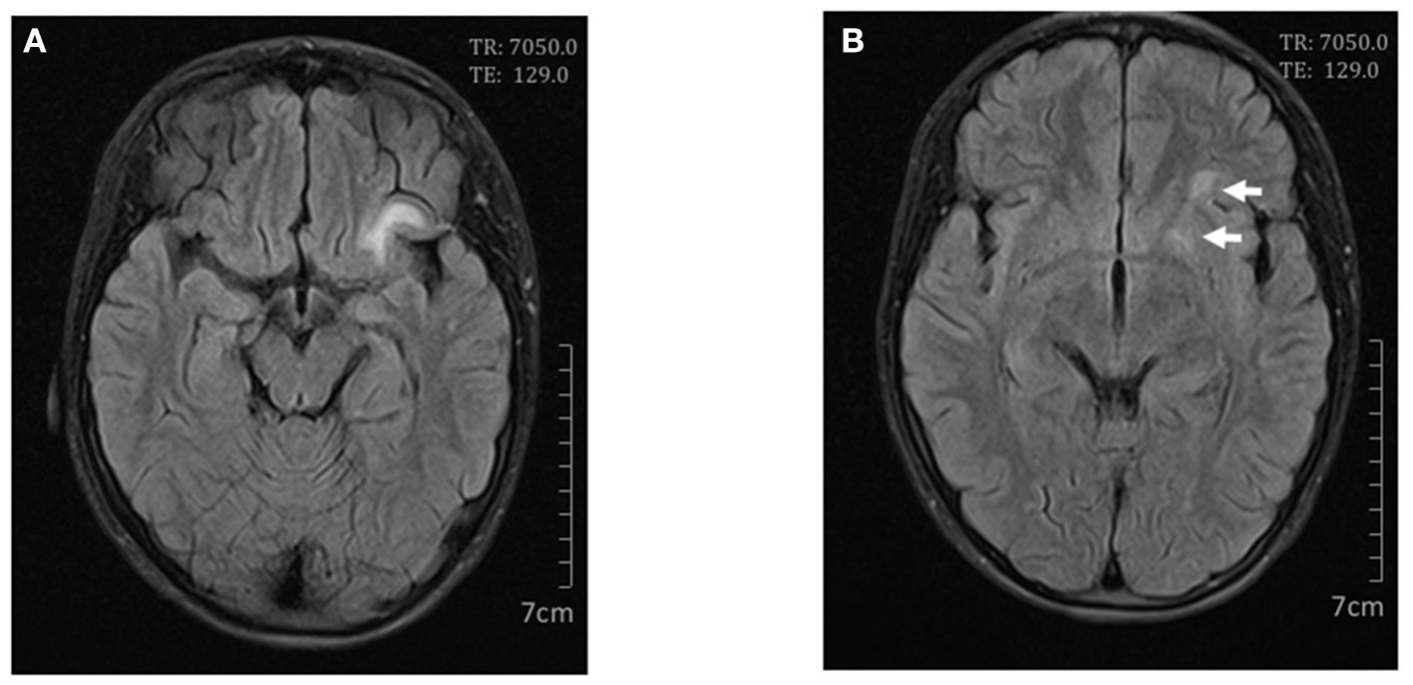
Cerebral magnetic resonance imaging on day 4. Axial FLAIR scan showed high signals in the left frontal lobe (A) and lentiform nucleus (B).
